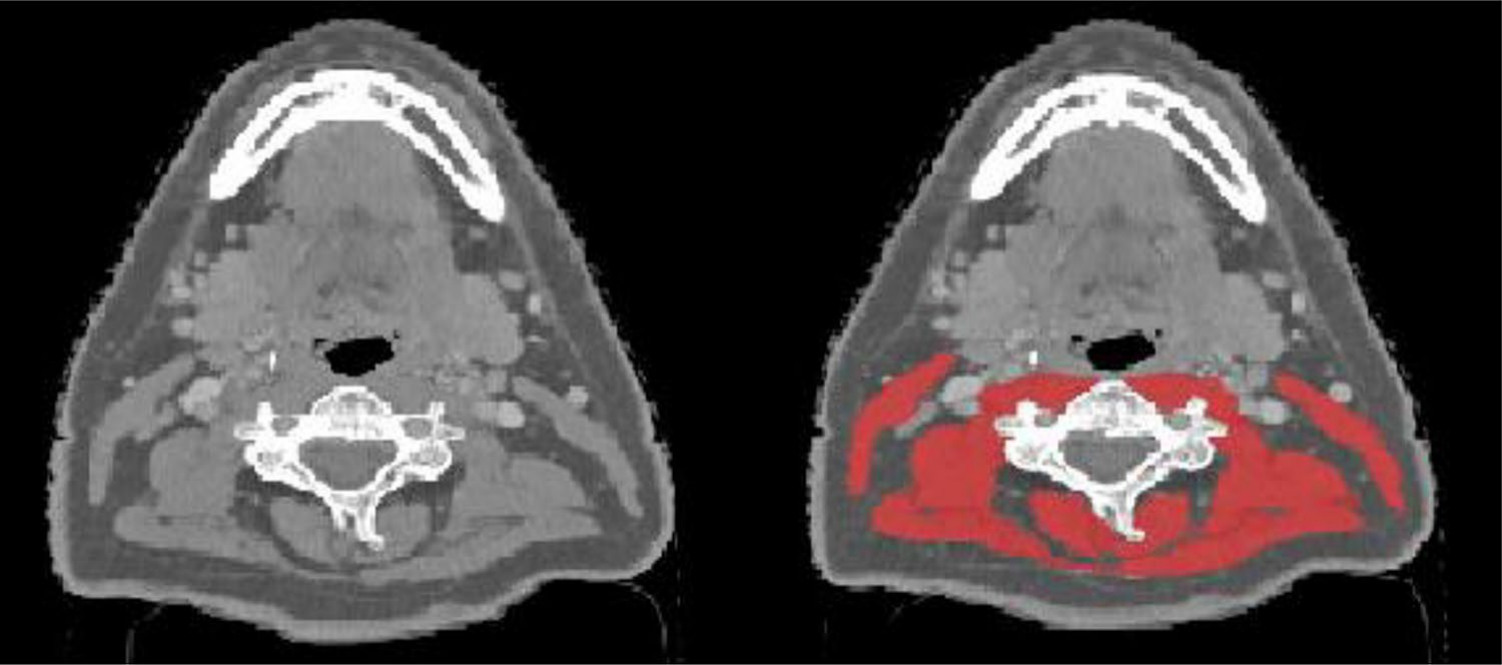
Example of segmentation of skeletal muscle tissue at the level of the third cervical vertebra (C3). Two identical axial contrast enhanced computed tomography (CT) slides at the level of C3; left shows the muscle tissue unsegmented, right shows both sternocleidomastoid and paravertebral muscles segmented in red
